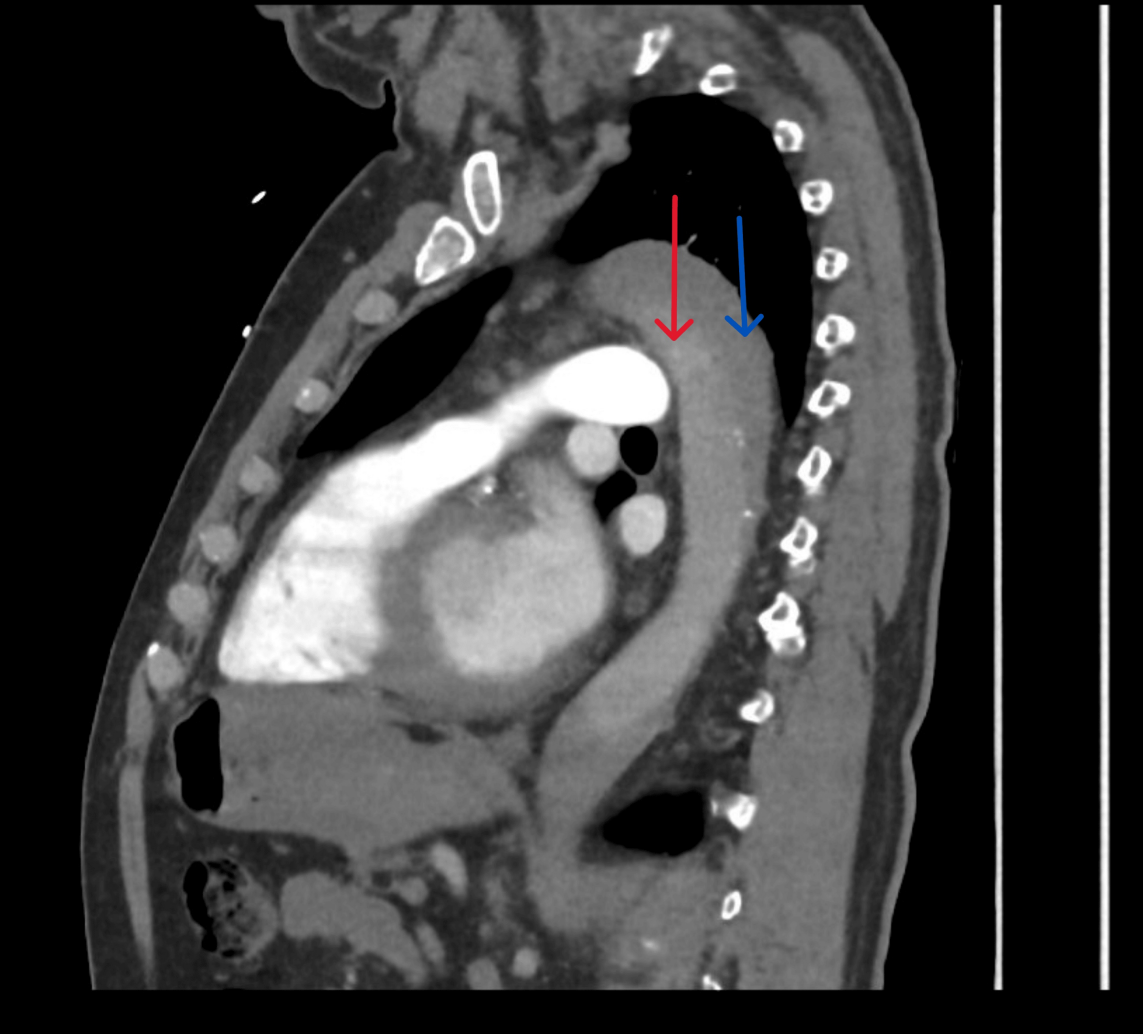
CTPA in sagittal view. Computed tomography pulmonary angiogram (CTPA) in sagittal view showing the dissected aorta. There is no contrast opacifying the aorta because this computed tomography (CT) scan was performed as a dedicated pulmonary embolism study, with contrast timing optimised for the pulmonary arterial circulation. Red arrow: true lumen; blue arrow: false lumen.
